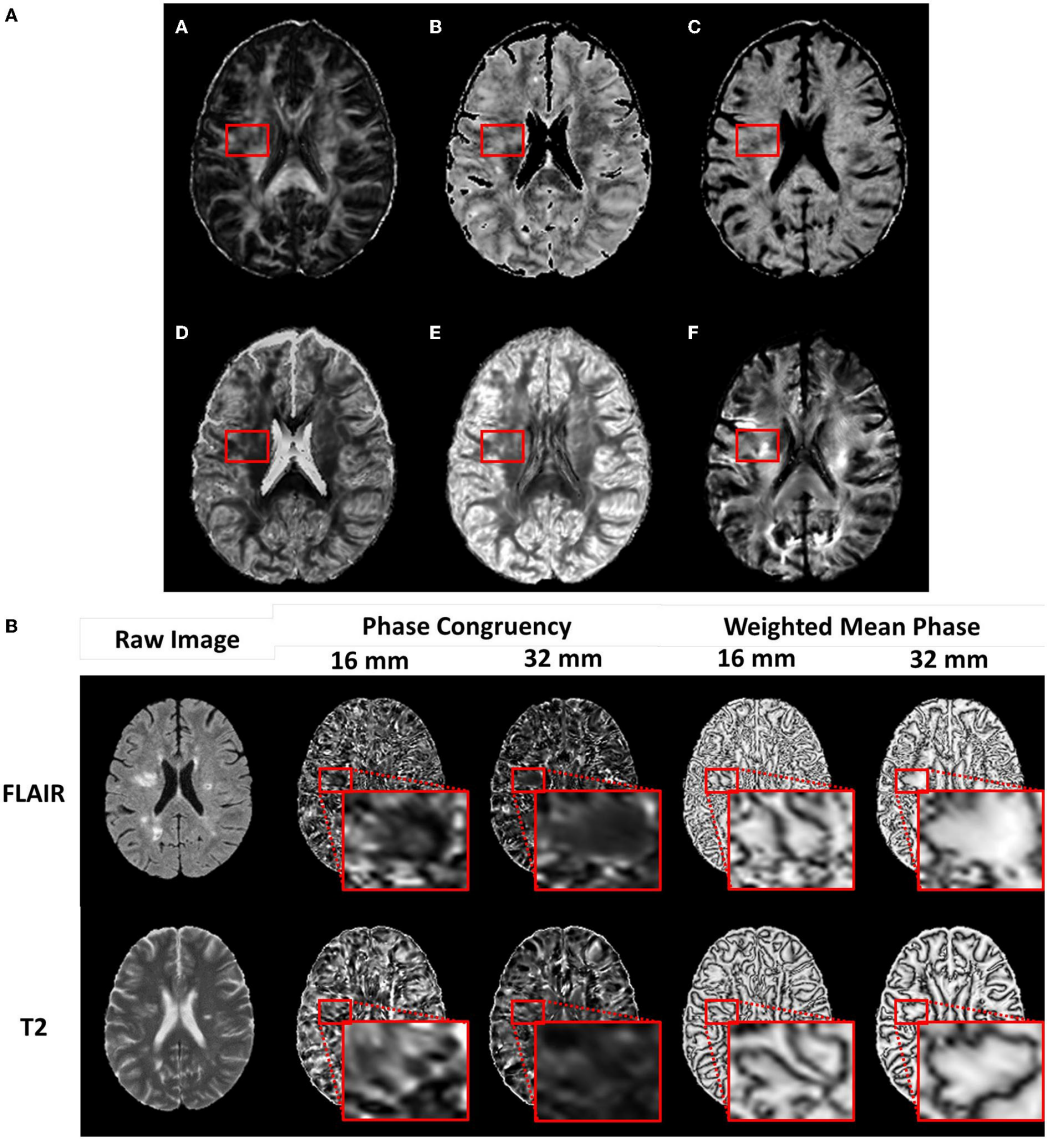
Example diffusion and phase congruency texture maps. (A) Diffusion MRI of a) fractional anisotropy, b) axonal diameter, c) intracellular volume fraction, d) Orientation Dispersion Index, e) Orientation Distribution Function energy f) Apparent Fiber Density, and (B) phase congruency of (top row) FLAIR MRI and (bottom row) T2 MRI.
